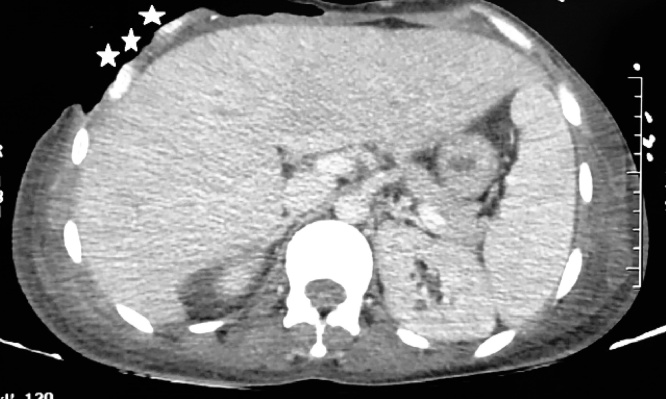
CT scan of the chest showing right-sided pleural effusion with erosive aspect of the ribs (white stars).
